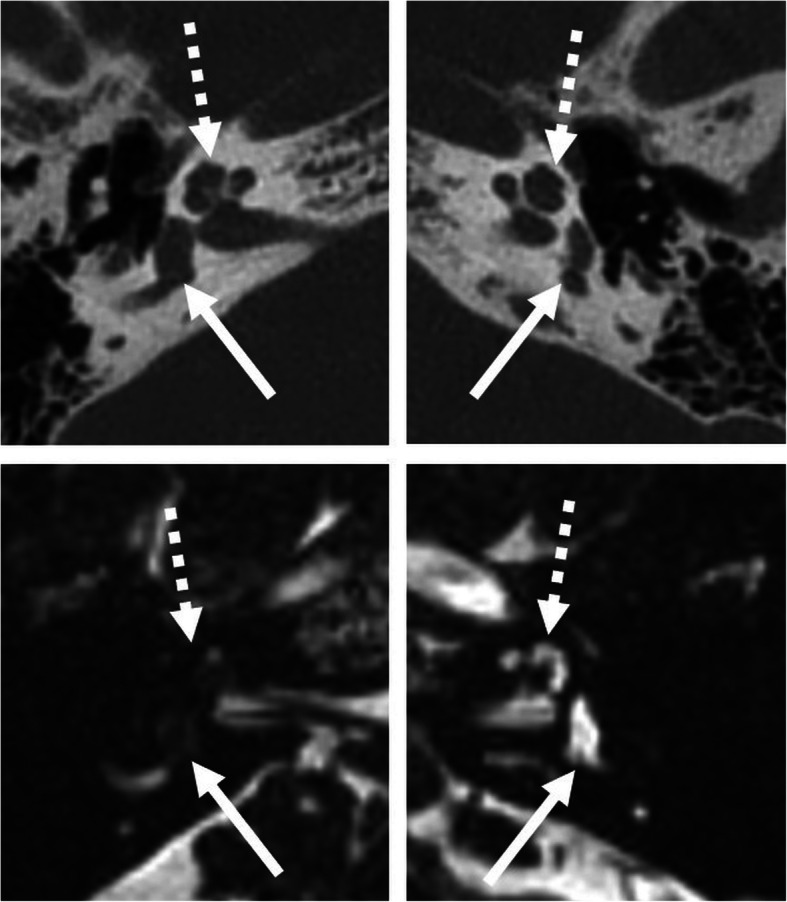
A 51-year-old male patient, with unilateral SNHL from several years. HRCT axial images (upper image) shows normal bony cochlea (dashed arrow) and vestibulum (arrow) on both sides. However, MRI 3D-CISS axial images (lower images) show loss of signal intensity of the right cochlea (dashed arrow) and vestibulum (arrow), compatible with fibrosis in early-stage labyrinthitis ossificans
